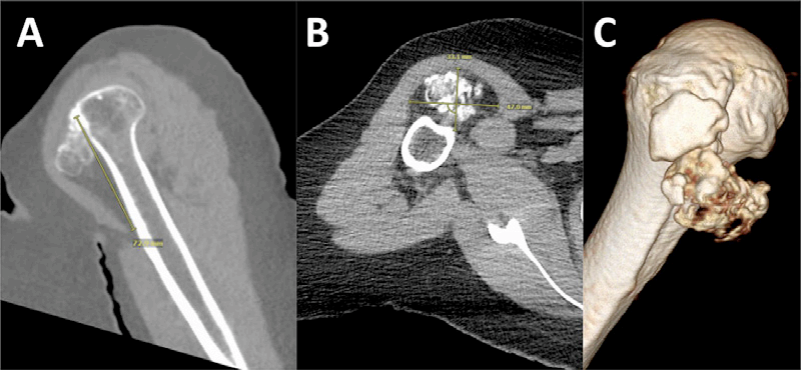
(A) Coronal, (B) Axial CT, and (C) 3D Reconstruction helical axial acquisitions encompassing the right shoulder without intravenous contrast.
